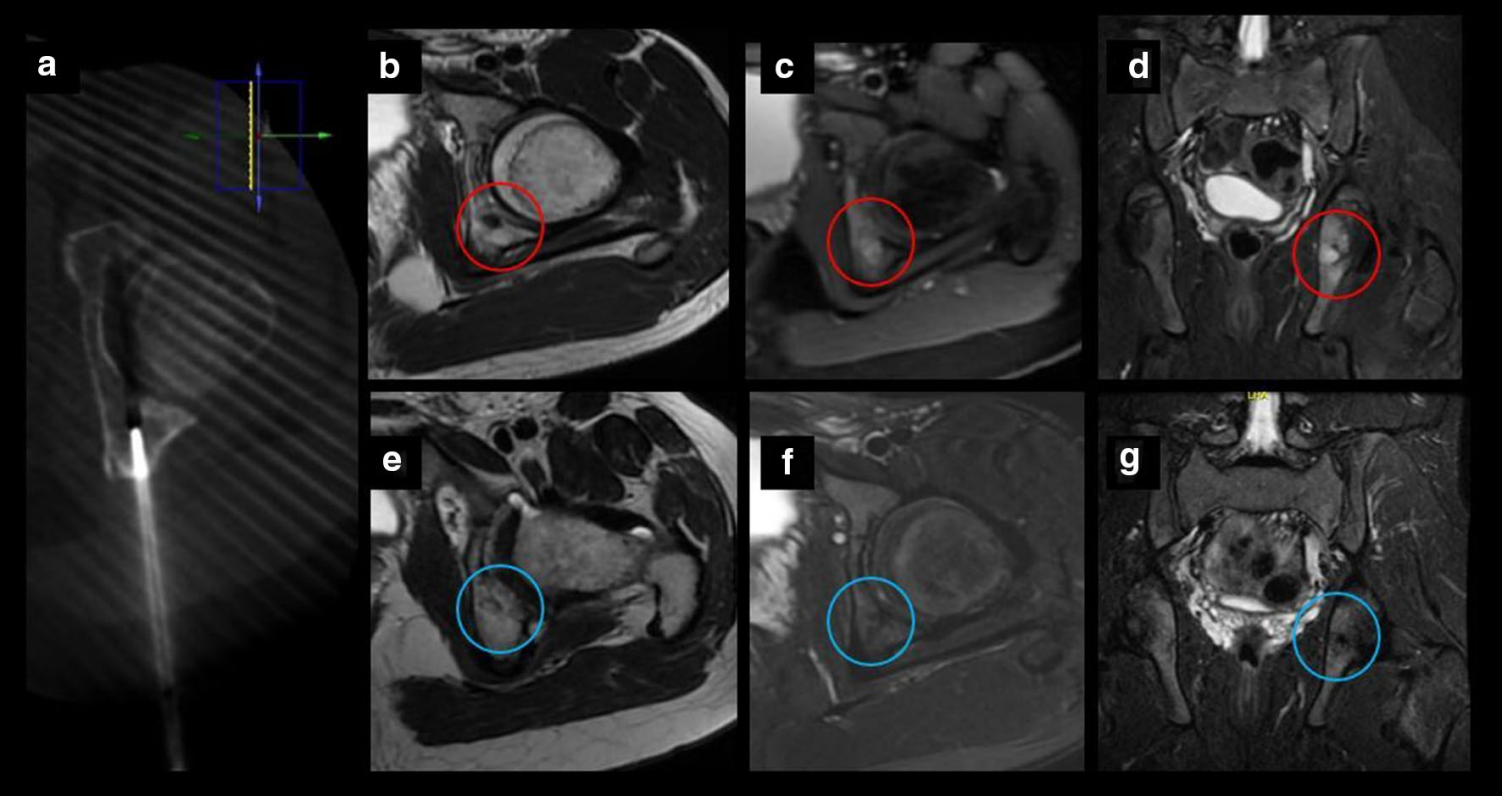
Chondroblastoma in a 14-y.o patient. a Axial CT exhibiting one cryoprobe placed within the lesion. b–d MRI images demonstrating a small tumor in the left posterior acetabulum corresponding to chondroblastoma (red circle). e–g Follow-up MRI images 20 months after treatment with no evidence of recurrence (blue circle)
